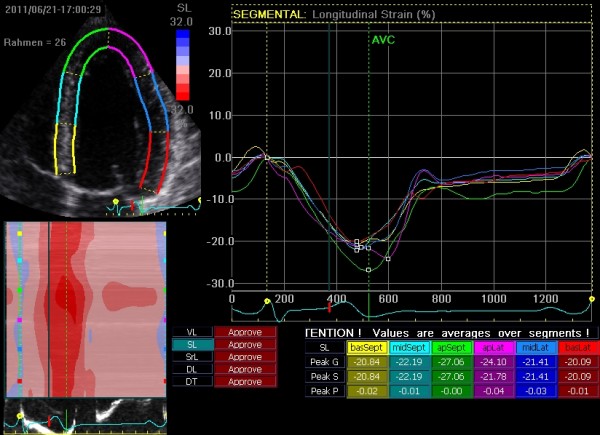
2D-Strain analysis of the left ventricle in the apical two chamber view with average values for peak systolic strain (Peak S) peak global strain (Peak G) and peak positive strain (Peak P) over each segment.
